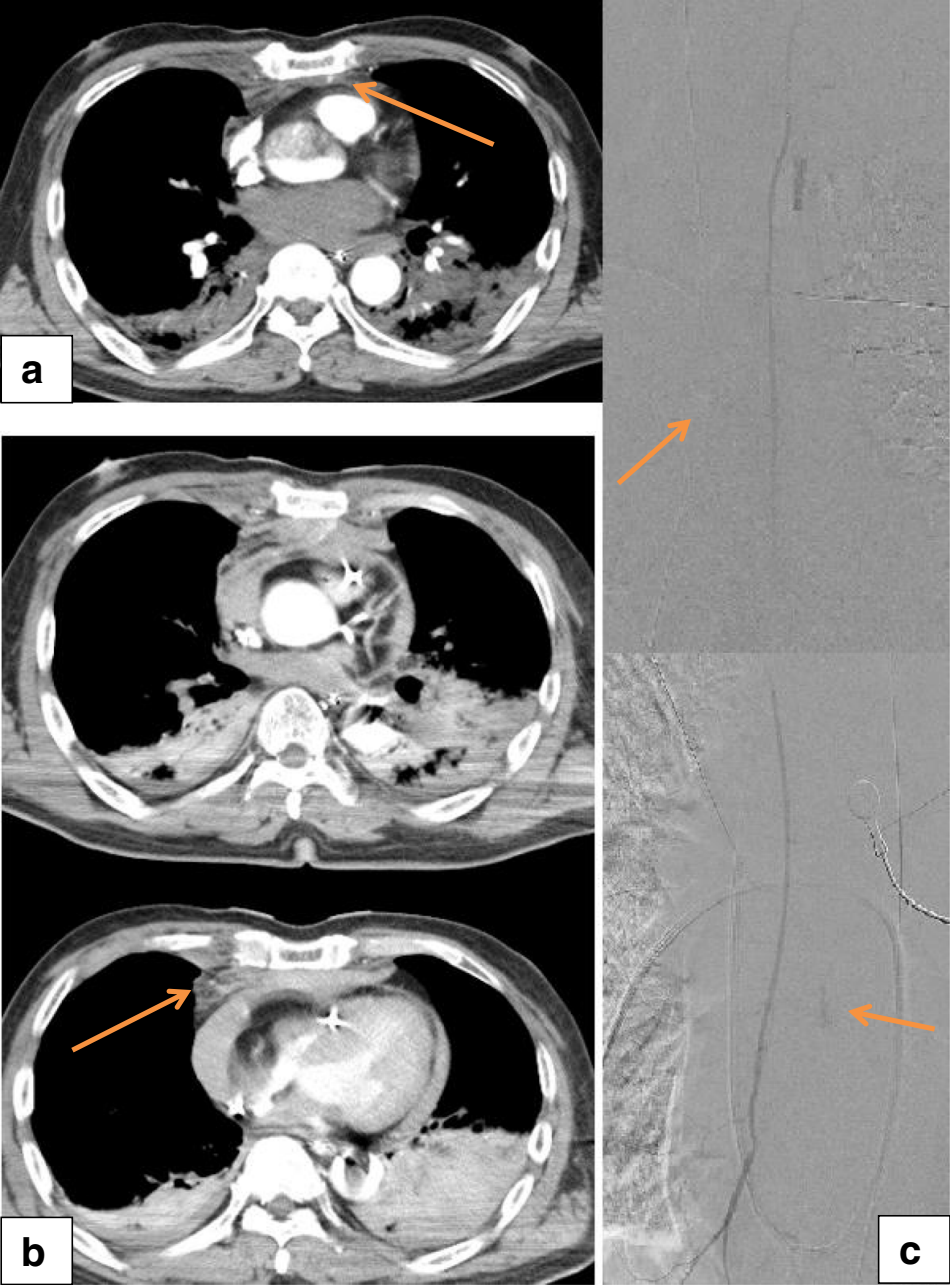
Computed tomography and angiography findings from Patient 4. a Contrast-enhanced computed tomography before the coronary angiography revealed a sternal fracture and a retrosternal hematoma with contrast extravasation of contrast (*arrow*). b Repeat computed tomography after the percutaneous coronary intervention revealed new contrast extravasation behind the fifth costal cartilage and beside the sternum (*arrow*), as well as growing hematomas in the retrosternal space. c Angiography after the percutaneous coronary intervention revealed bilateral contrast extravasation at the bilateral internal mammary arteries (*arrow*s)
